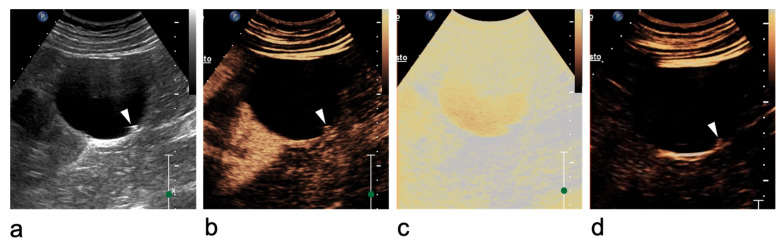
Ultrasound artefact mimicking an intracystic vegetation within a renal cyst at the lower pole of the right kidney. (a) Greyscale US shows focal thickening of the cystic wall (arrowhead). (b) On CEUS, enhancement of the focal thickening (arrowhead) is equivocal. (c) A high-mechanical index (MI) burst is applied to destroy microbubbles and determine whether the focal wall abnormality is vascularised. (d) After microbubble disruption, no change is observed (arrowhead), confirming the absence of vascularity. The wall thickening is therefore consistent with amorphous/intraluminal material rather than an enhancing vegetation (Bosniak category II cyst).
